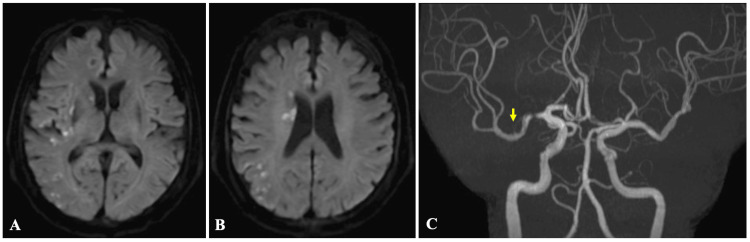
(A and B) Brain magnetic resonance imaging and (C) angiography at day 2 Axial diffusion-weighted images show no new hyperintense lesions and stable findings in the M1 segment of the middle cerebral artery territory (A and B). Magnetic resonance angiography demonstrates preserved patency in the M1 segment of the right middle cerebral artery, indicating maintained recanalization (C, arrow)
